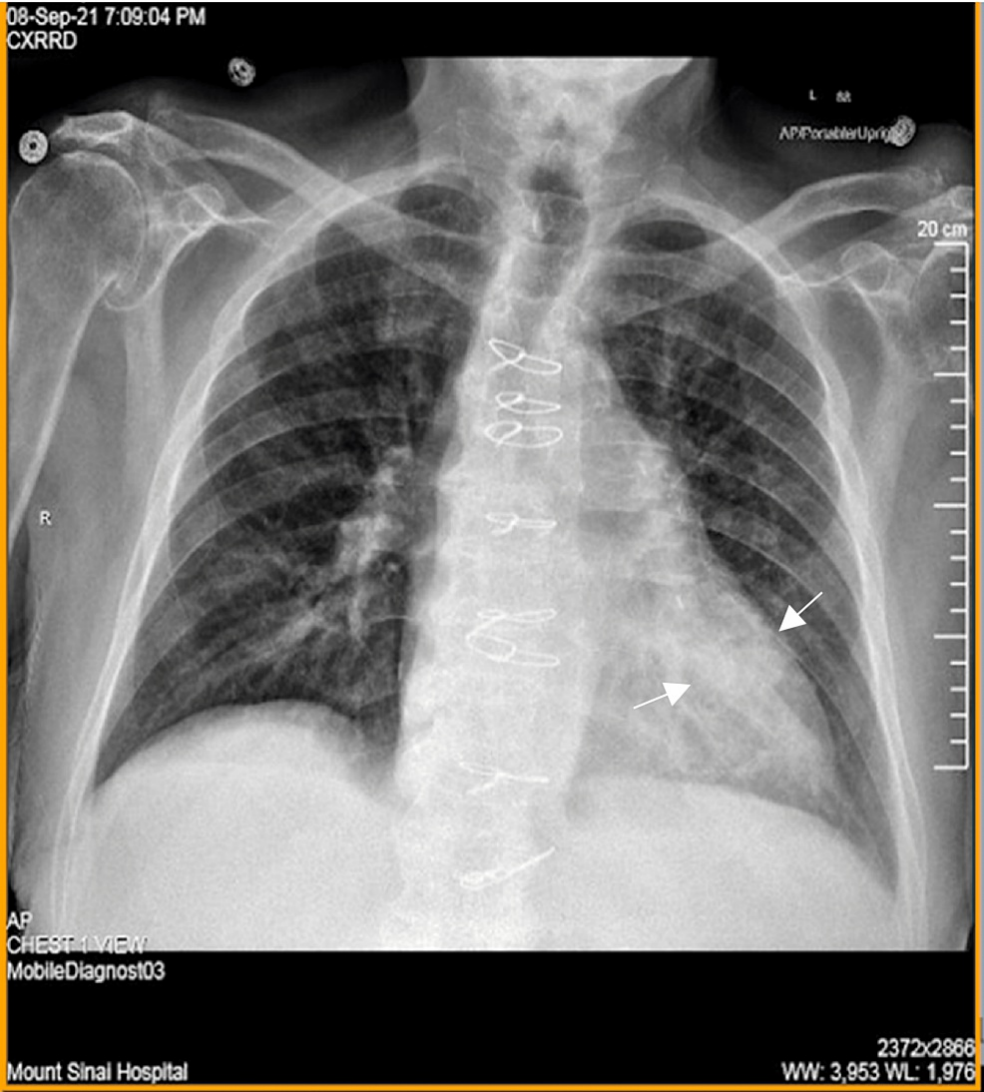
Chest radiograph on admission The opacity at the left retrocardiac region (white arrows) is suspicious for left lower lobe pneumonia versus atelectasis
